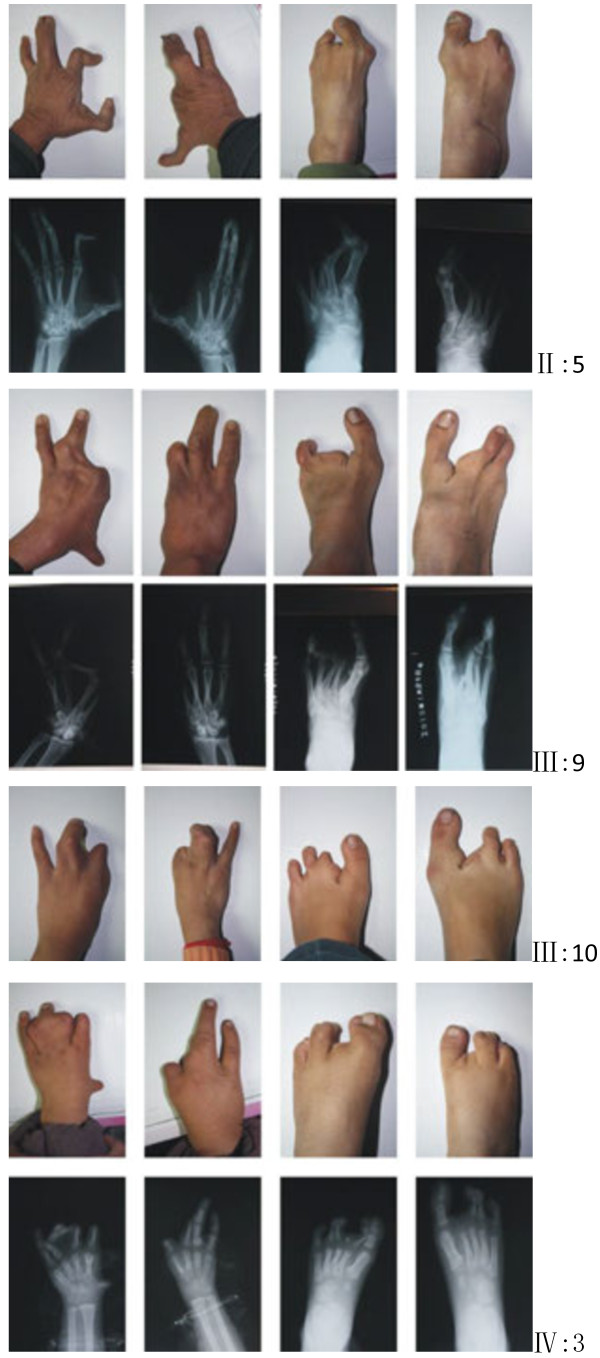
Clinical and X-ray photos of selected patients from this SHFM family.
